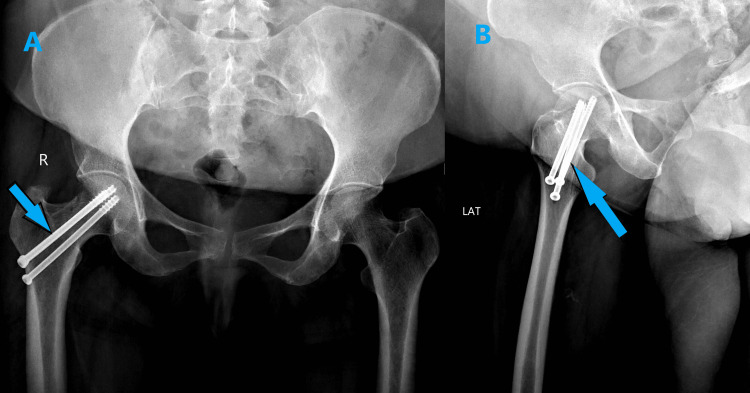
Immediate postoperative radiograph showing right femur neck stress fracture fixed with cannulated hip screw (A&B)
